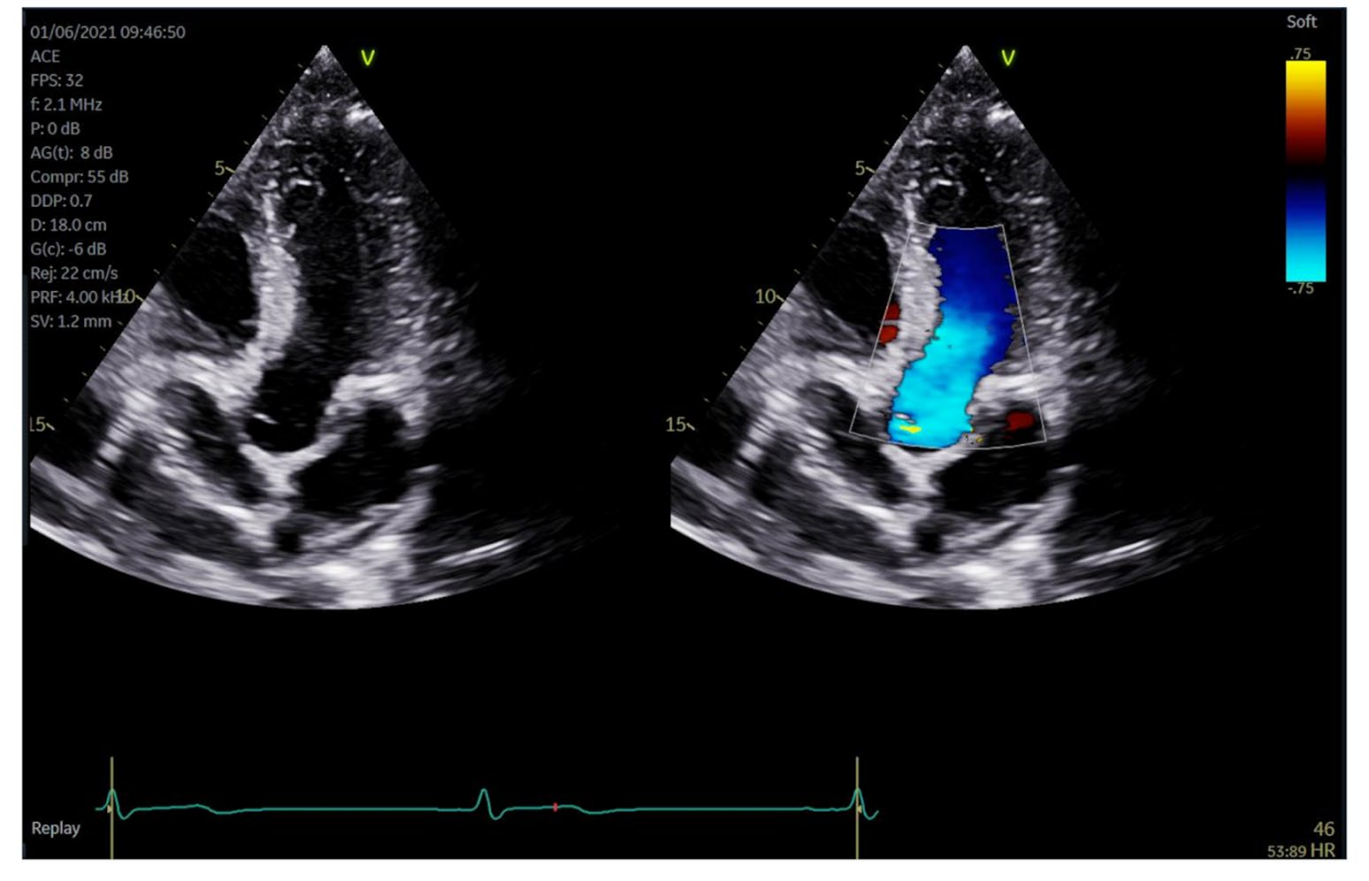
Simultaneous Colour Doppler Imaging. An apical 5 chamber view demonstrates simultaneous colour mode where both the 2D and colour Doppler images are displayed together in real time
